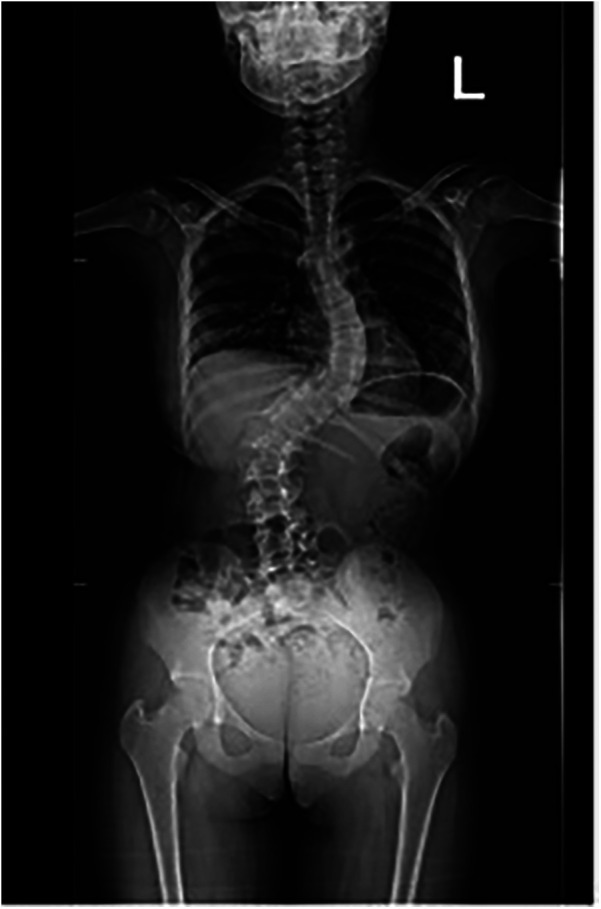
Preoperative anteroposterior radiograph showing severe neuromuscular scoliosis.
